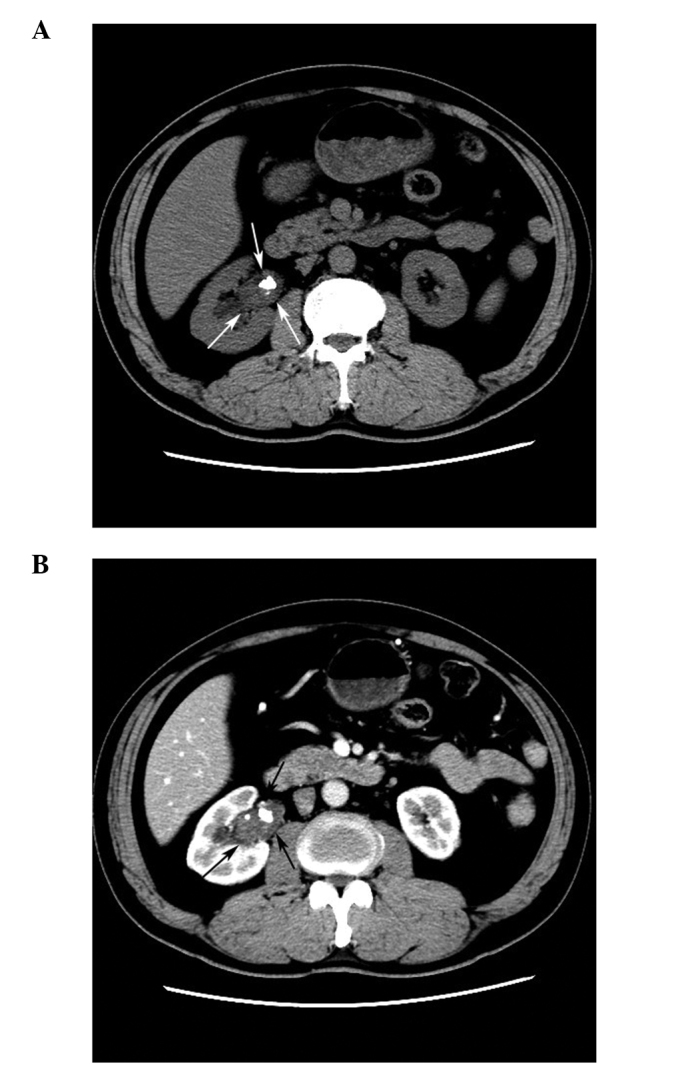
(A) Plain computed tomography (CT) scan. White arrows indicate a 2.0×1.6×3.4-cm mass with a central high density in the right renal pelvis. (B) Contrast-enhanced CT scan. Black arrows indicate that the mass was marginally enhanced in the arterial phase.
